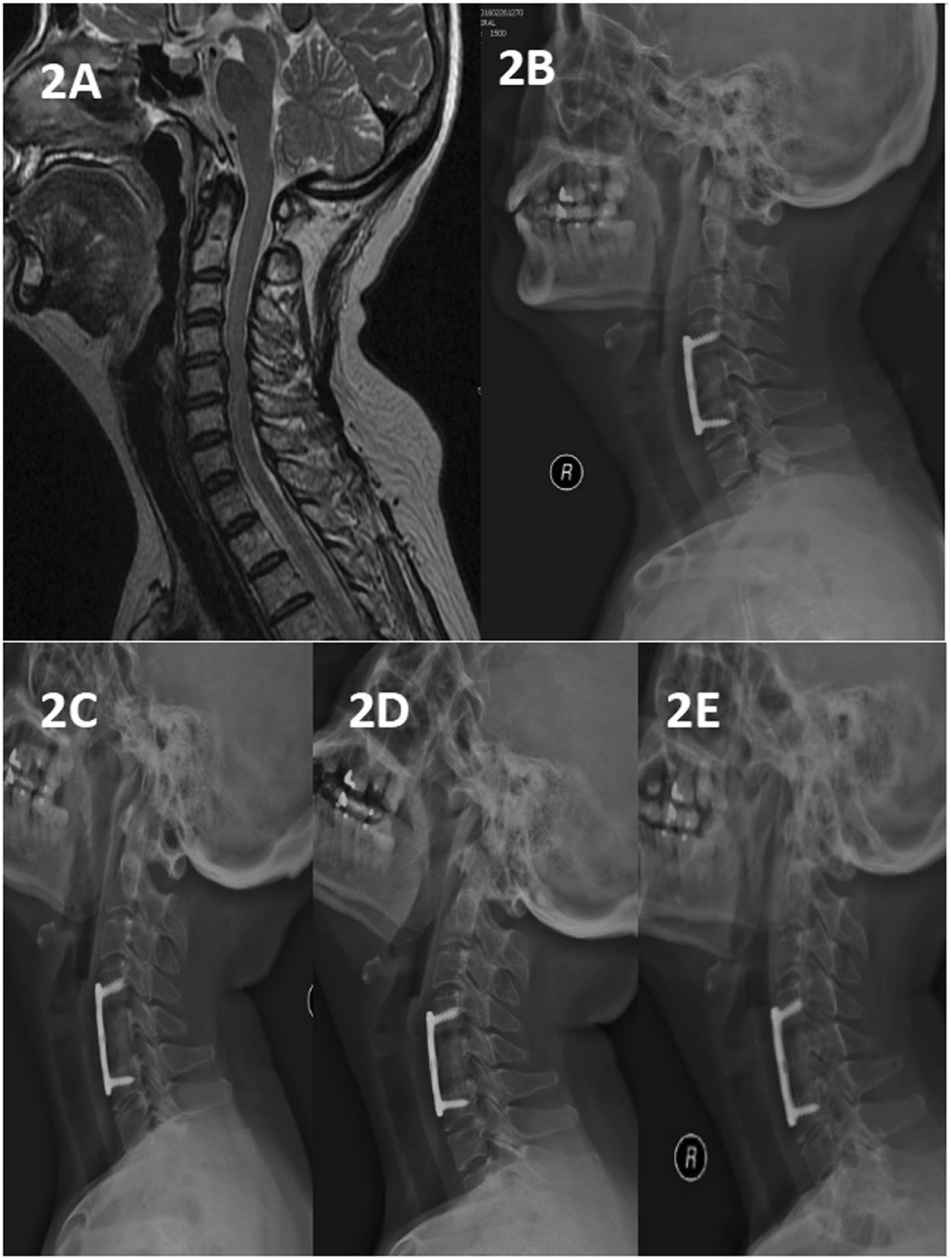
A 50-year-old woman who underwent 1-level corpectomy with a nanohydroxyapatite/polyamide66 strut for cervical reconstruction. The preoperative cervical MRI (A) revealed C4/5 disc herniation. The patient underwent a C5 corpectomy and fusion using an n-HA/PA66 strut (B). The lateral X-ray film indicated that the autogenous bone granules filling the strut had achieved bony fusion, and subsidence was observed at the 3-month follow-up (C). The strut and internal fixation were in position at 3, 6, and 12 months of follow-up (C–E).
